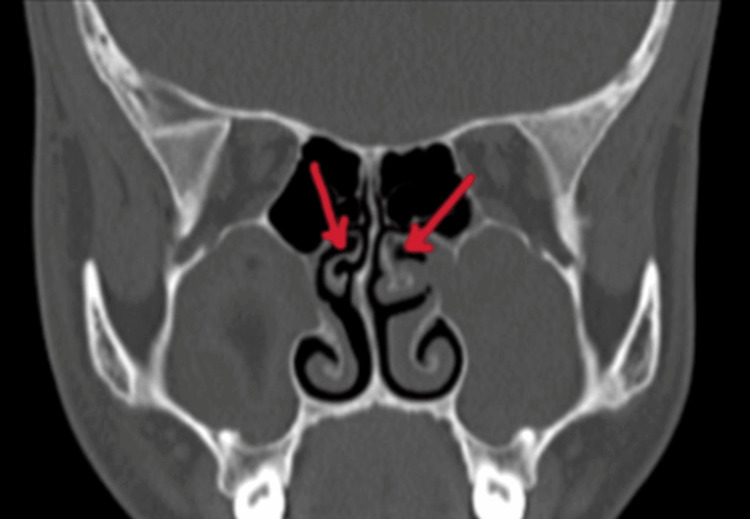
CT showing paradoxical curvature of middle turbinates (as indicated with red arrows).
